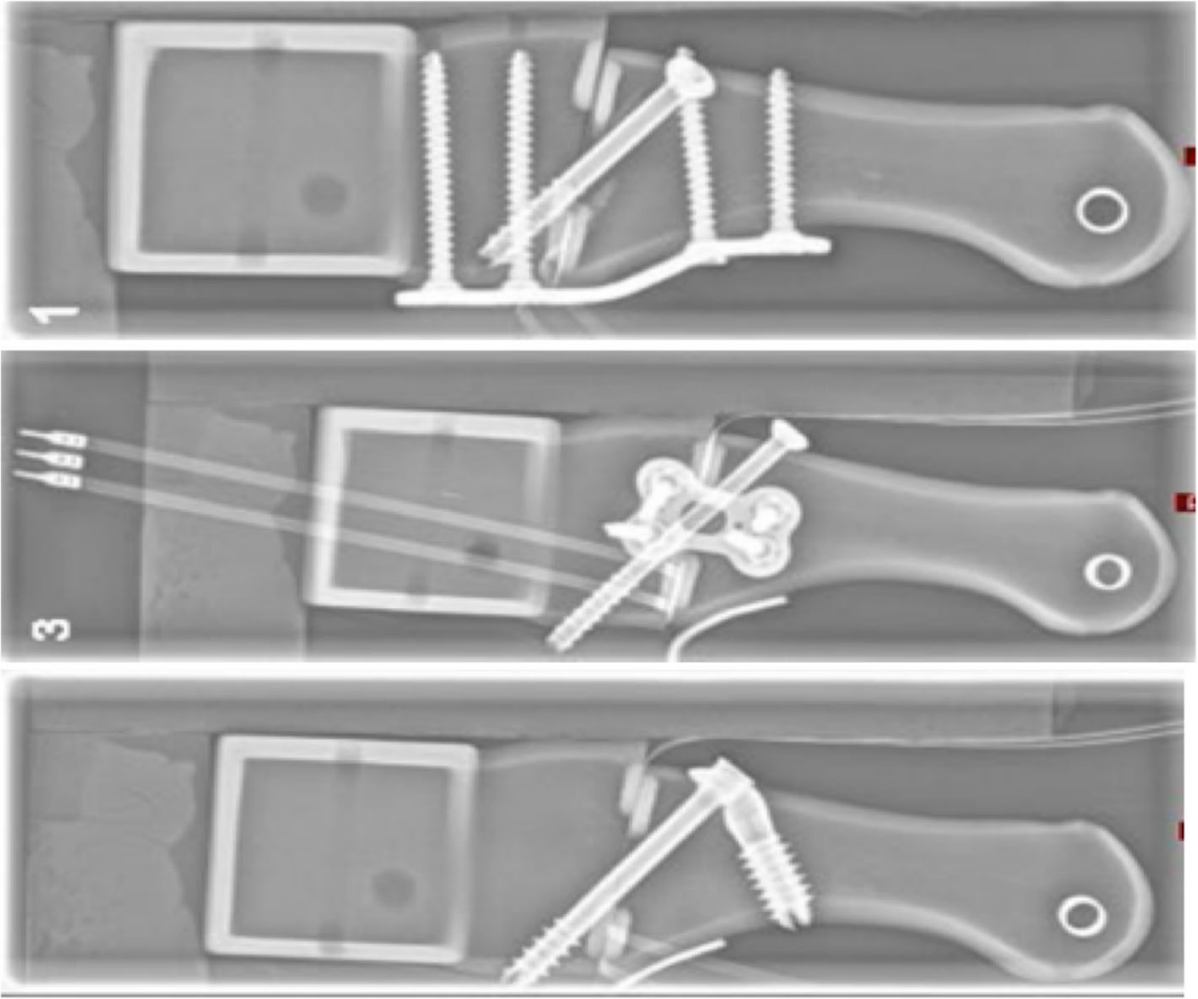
X-ray images of the three models. a Plantar locking plat with crossed screw, b medial locking plate with crossed screw, and c the intramedullary angle-stable implant
